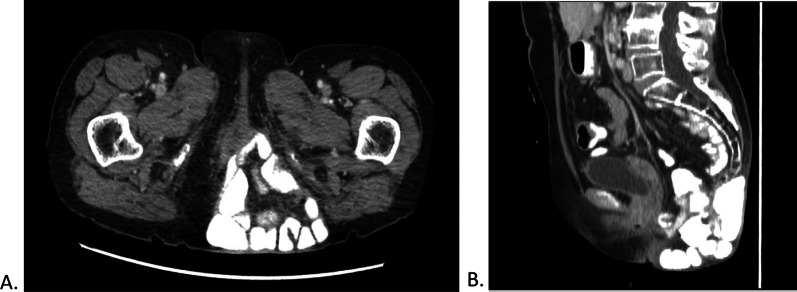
A CT-scan of pelvic outlet showing herniation of multiple, small intestine loops, with contrast material. Axial view. B Extent of herniation shown from a midsagittal view
